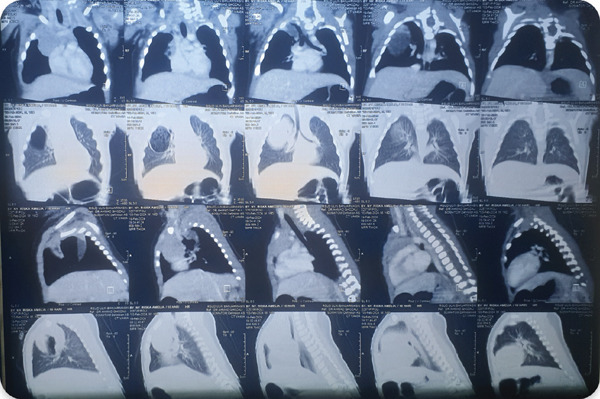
Mediastinal window of CT scan.
